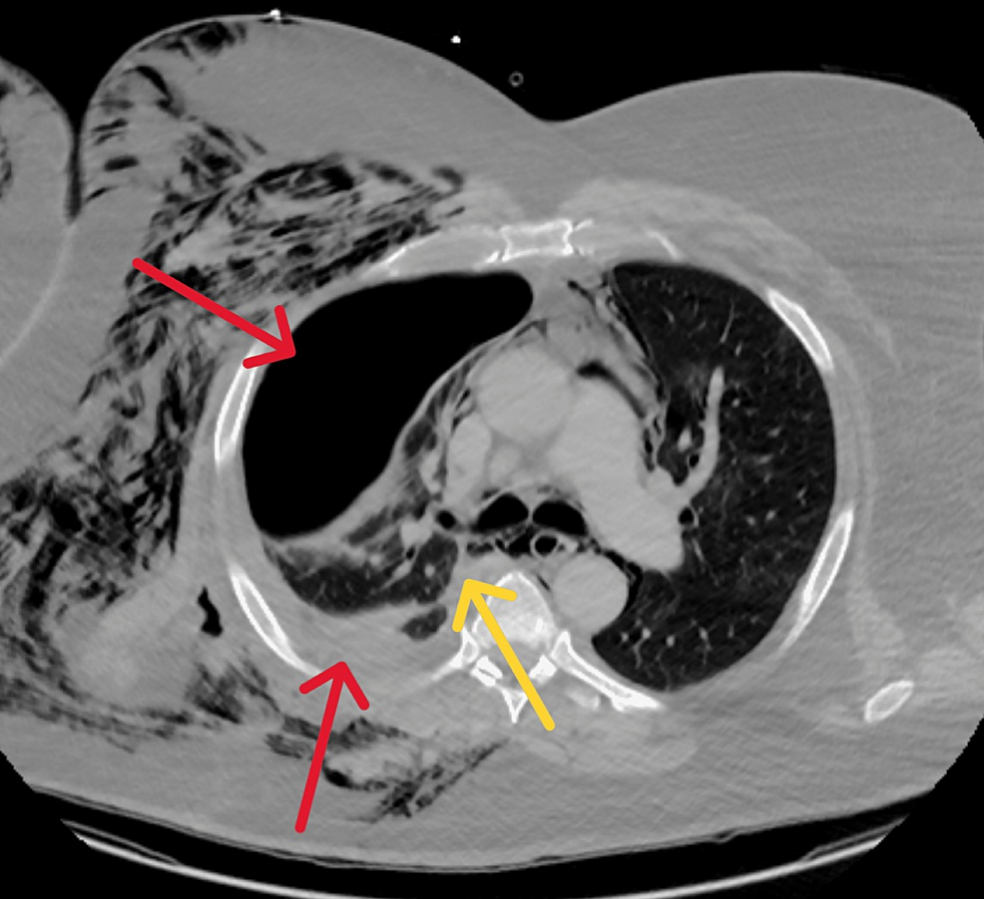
Computed tomography (CT) chest with contrast The red arrow shows a large right-sided hydropneumothorax comprising approximately 50% of the right hemithorax volume. The yellow arrow shows the development of pneumomediastinum.
